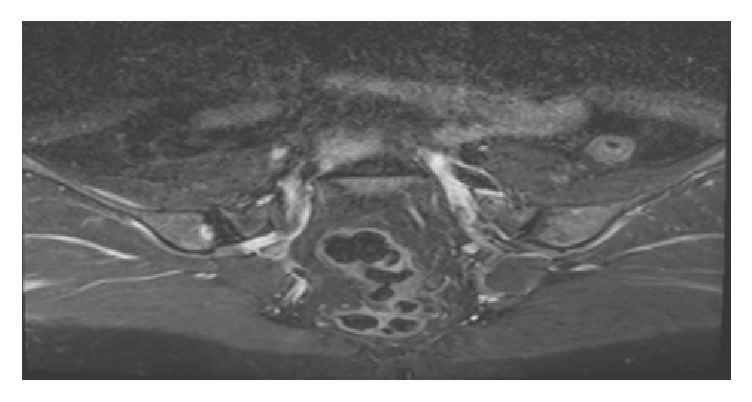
Signal enhancement consistent with the right iliac focal bone marrow edema adjacent to the right sacroiliac junction, T2 MRI sequences.
